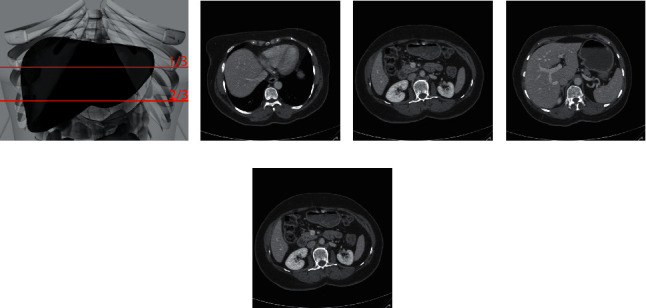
Frontal outline and the upper, middle, and lower sections of the liver CT scan sequence.
